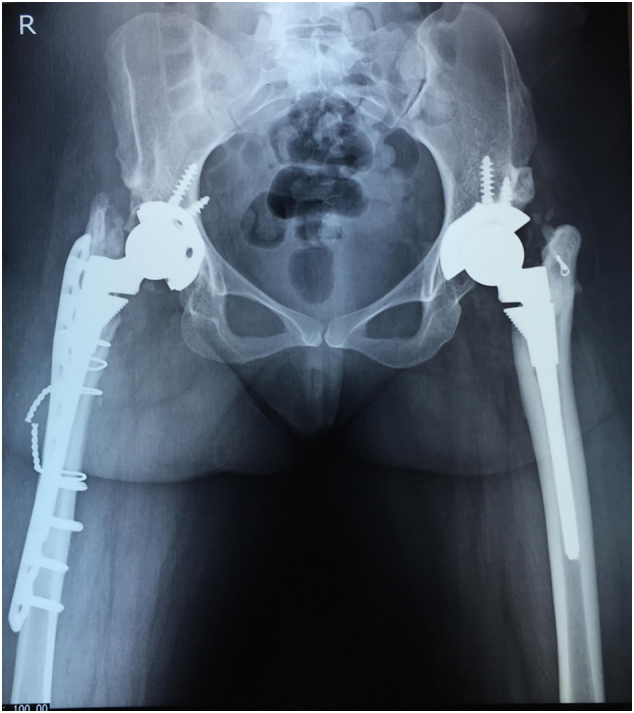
Post-operative X-ray after the second arthroplasty showing bilateral satisfactory reconstruction.
